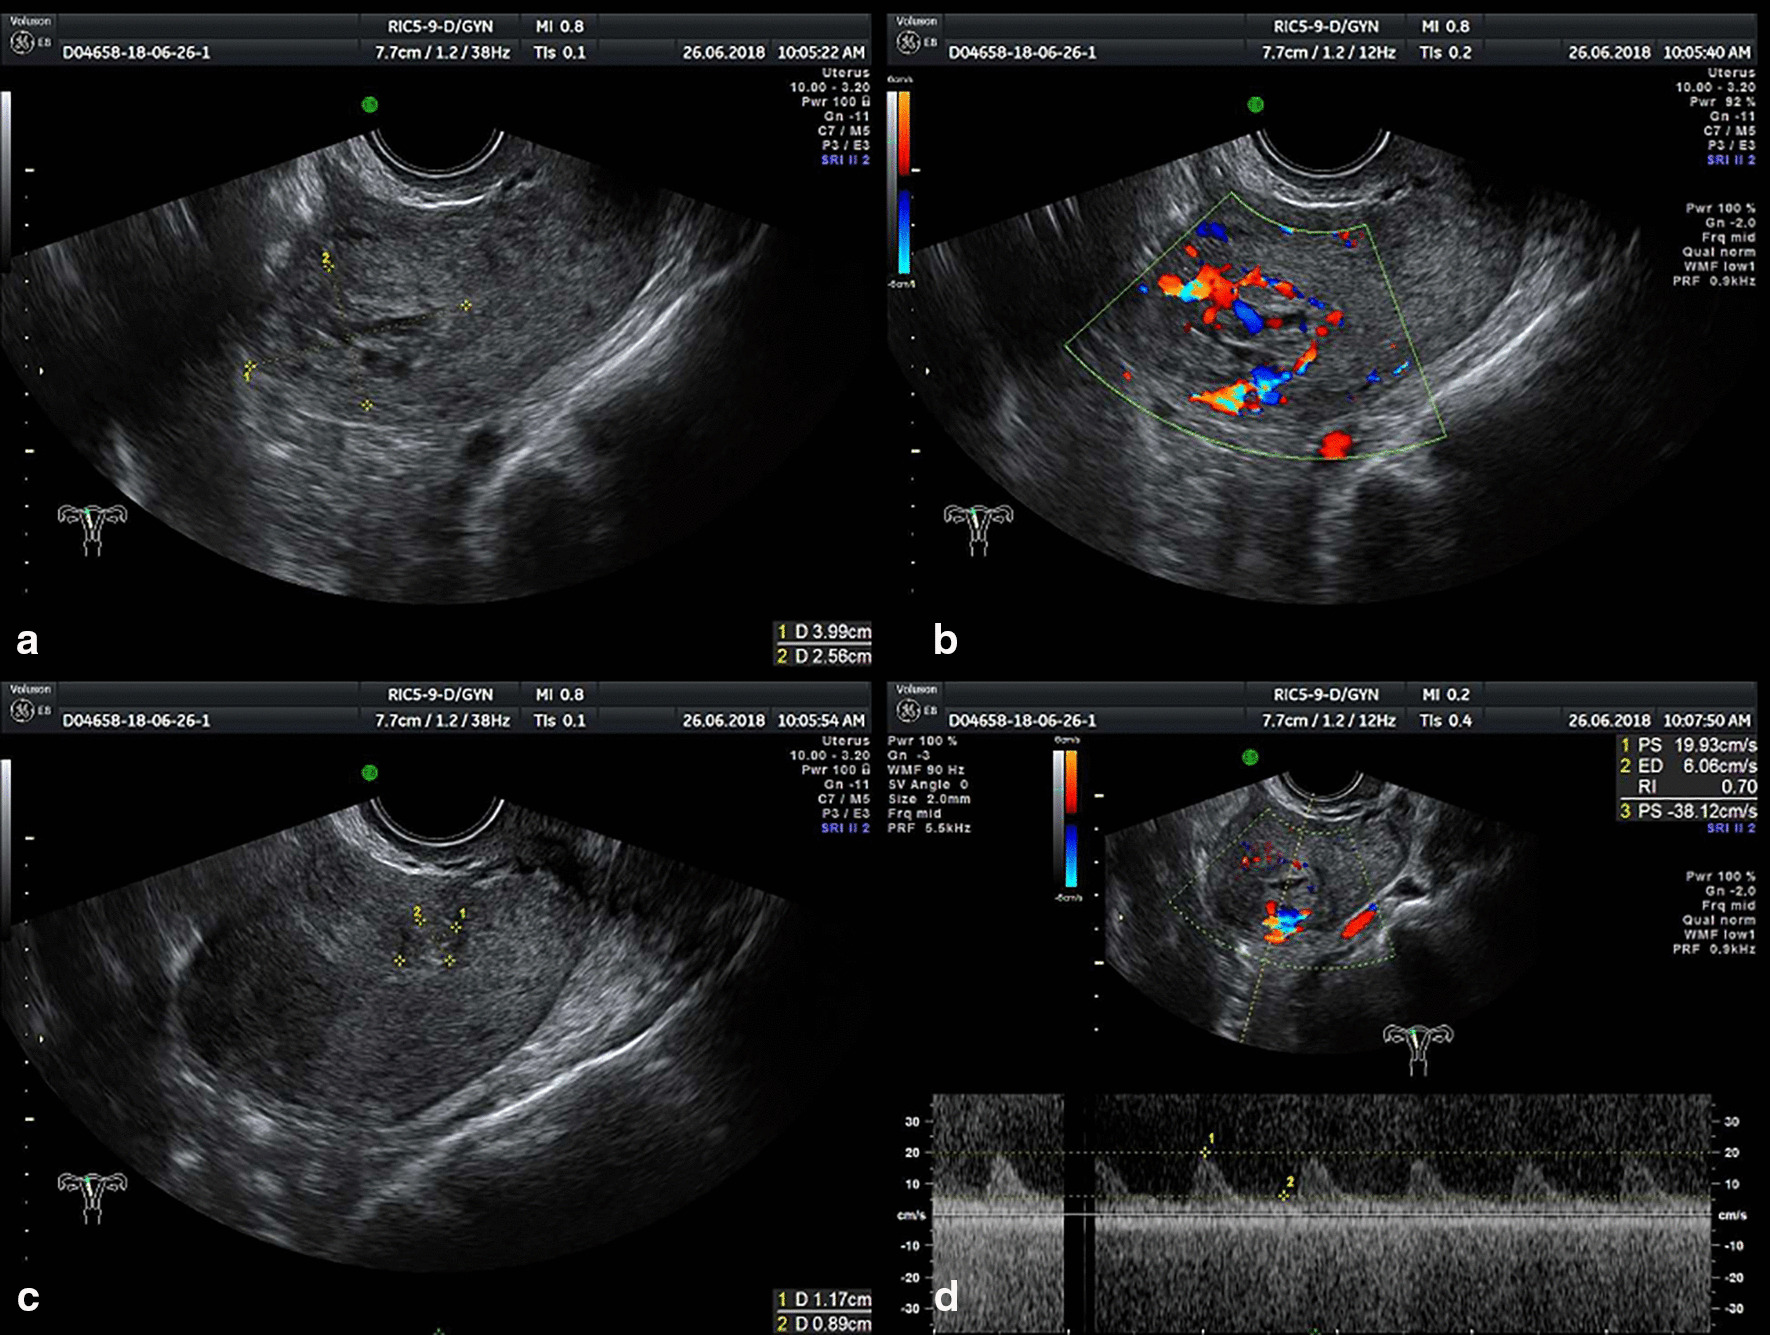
Endovaginal sonography image of case 2. “Honeycomb-resembling” disordered echoes with unclear border located in the left angular of the uterus measuring in 3.99 * 2.56 cm. In figure a and c, Gestation tissue is marked by yellow dots which was used to measure gestation tissue. In figure b and d, yellow measurement line circles gestational sac
